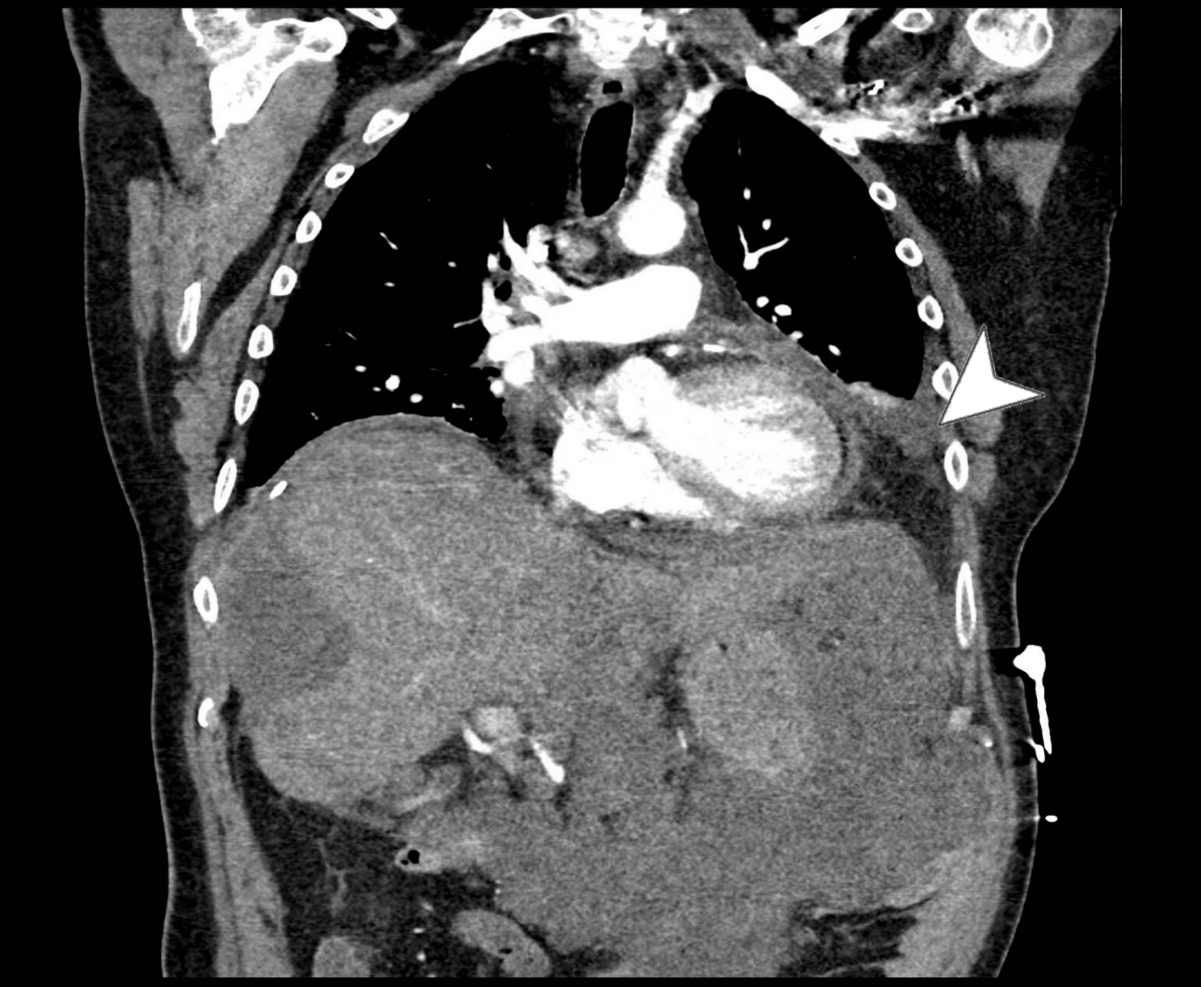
Coronal CT view showing left-sided organised pleural effusion
